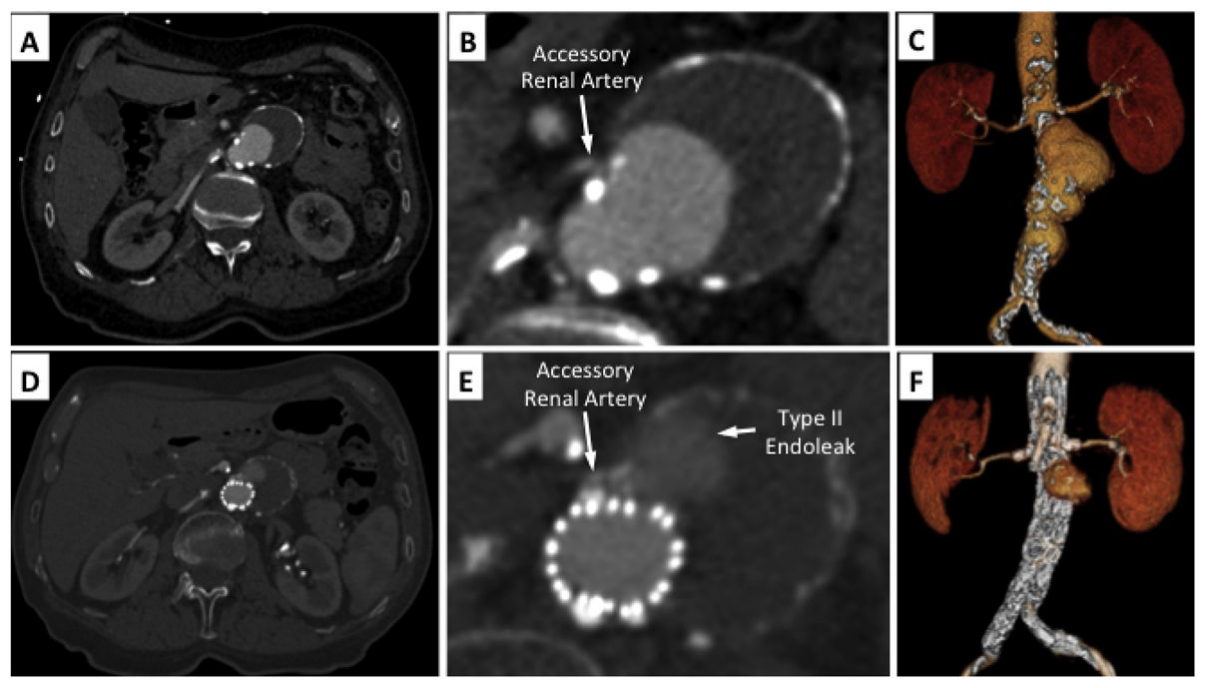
Type II endoleak from an accessory renal artery. (A–C) Preoperative CTA demonstrated a fusiform juxta-renal AAA. Cross-sectional inspection of CTA demonstrated a small accessory left renal artery. (D–F) Postoperative CTA demonstrated a well-positioned fenestrated aortic endograft with patent renal artery stents. A Type II endoleak is visualized directly adjacent to the accessory renal artery from delayed retrograde filling.
